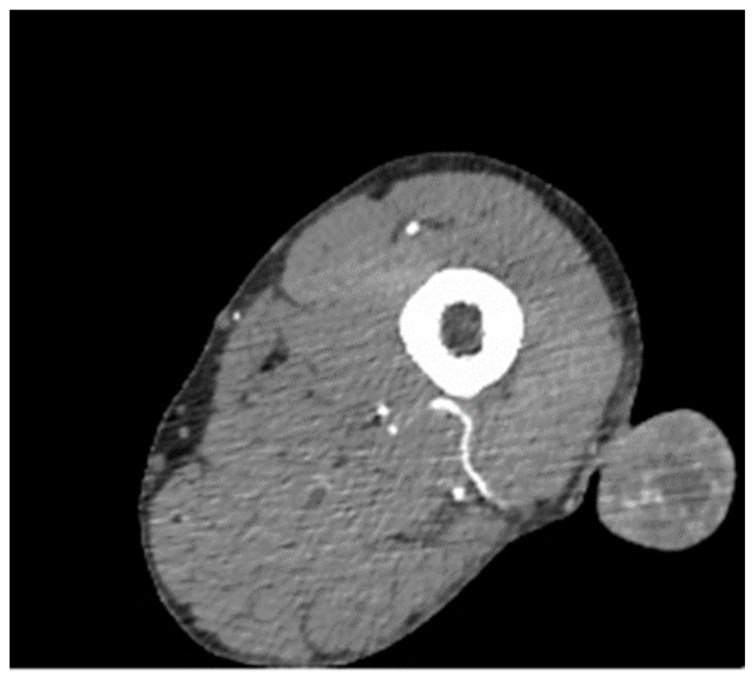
The CT angiographic scan of the lower limb shows the nodular formation with a vascular pedicle connected to the profound femoral artery. Blood vessels are also noticed inside (Supplemental Figure S1).
